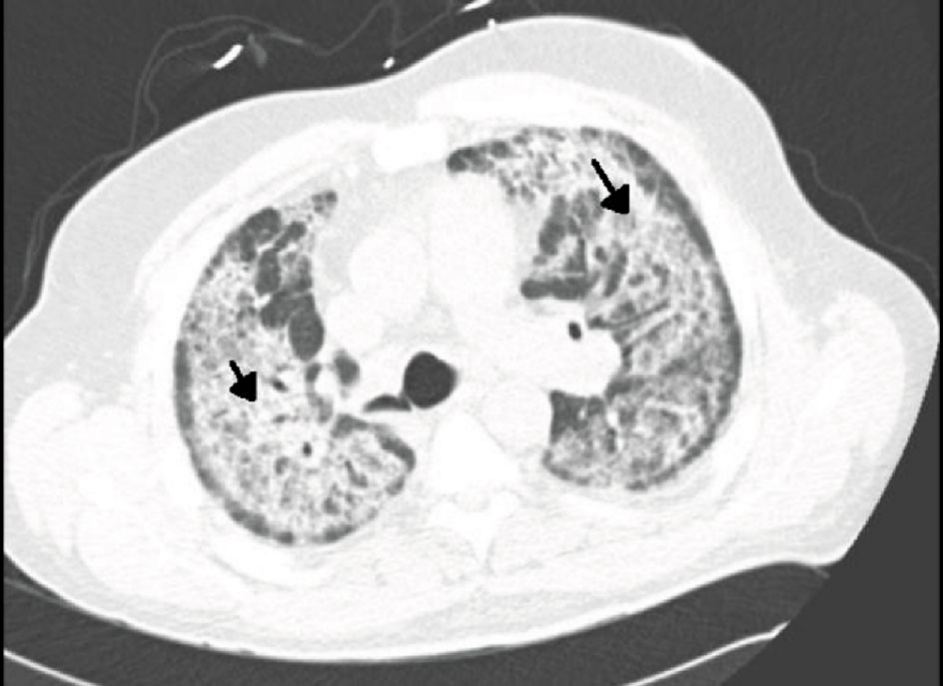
CT of the thorax showing extensive bilateral infiltrates and ground glass type densities (black arrow) sparing the periphery.
